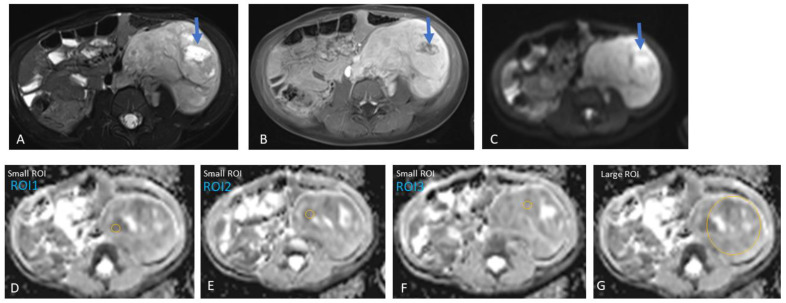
Left retroperitoneal neuroblastoma with areas of necrosis in a three-year-old girl. Heterogeneous tumour, predominantly solid with a focal fluid area (arrow), potentially representing necrosis within the tumour. Therefore, this focal area of fluid was avoided during the placement of the regions-of-interest (ROIs) in the tumour for data analysis. (A) Axial T2, (B) axial post-gadolinium T1, and (C) axial DWI (b-value: 800 mm^2^/s). (D–F) Three small ROIs on the ADC map with an area of 19.1 mm^2^ and an average value of 1.23 × 10^−3^ mm^2^/s, and (G) one large ROI with an average area of 2721.1 mm^2^ and an average value of 1.42 × 10^−3^ mm^2^/s.
